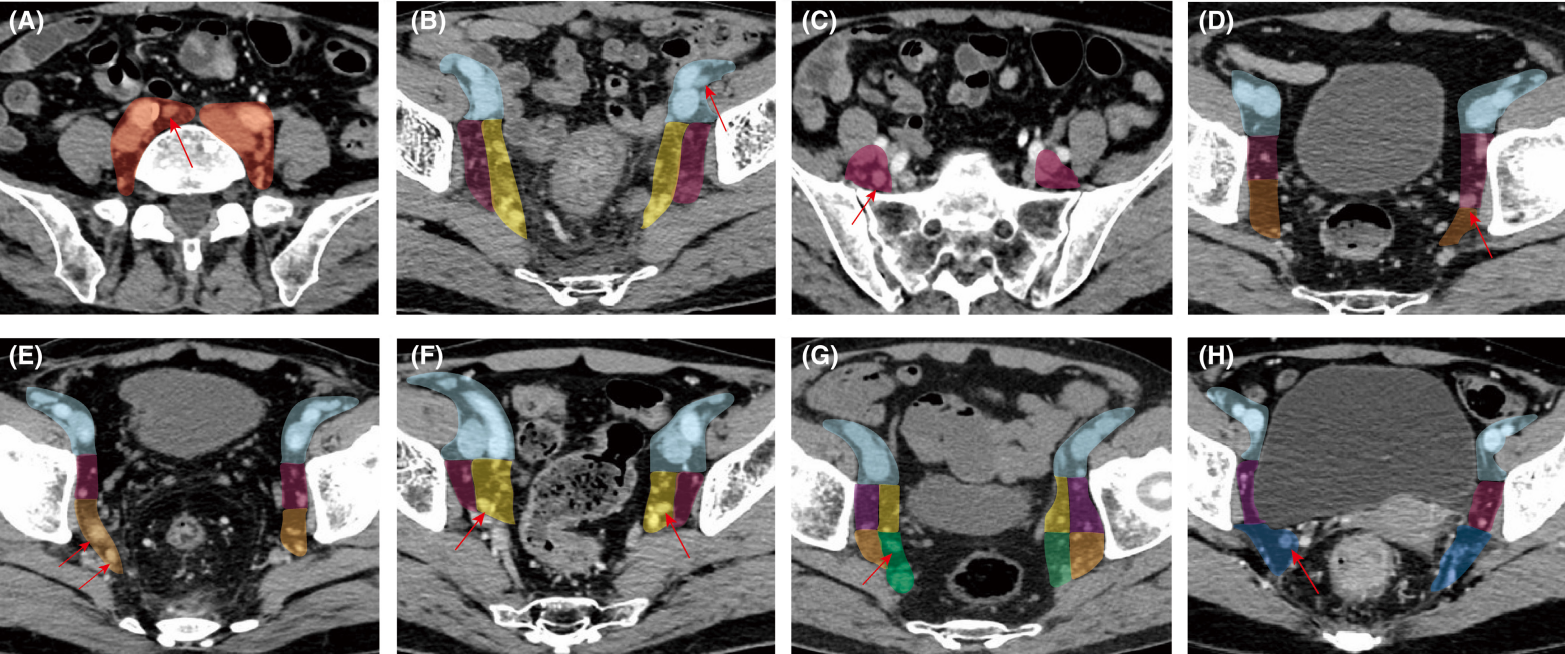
CT scan showed LLNs located the seven lateral compartments. (A) (light red), common iliac compartment; (B) (sky blue), external iliac compartment; (C, D) (purple), obturator cranial compartment; (E) (light orange), obturator caudal compartment; (F) (yellow): Proximal internal iliac compartment; (G) (light green): Distal internal iliac compartment; (H) (blue): Extended distal internal iliac compartment. Red arrow indicated LLNs.
